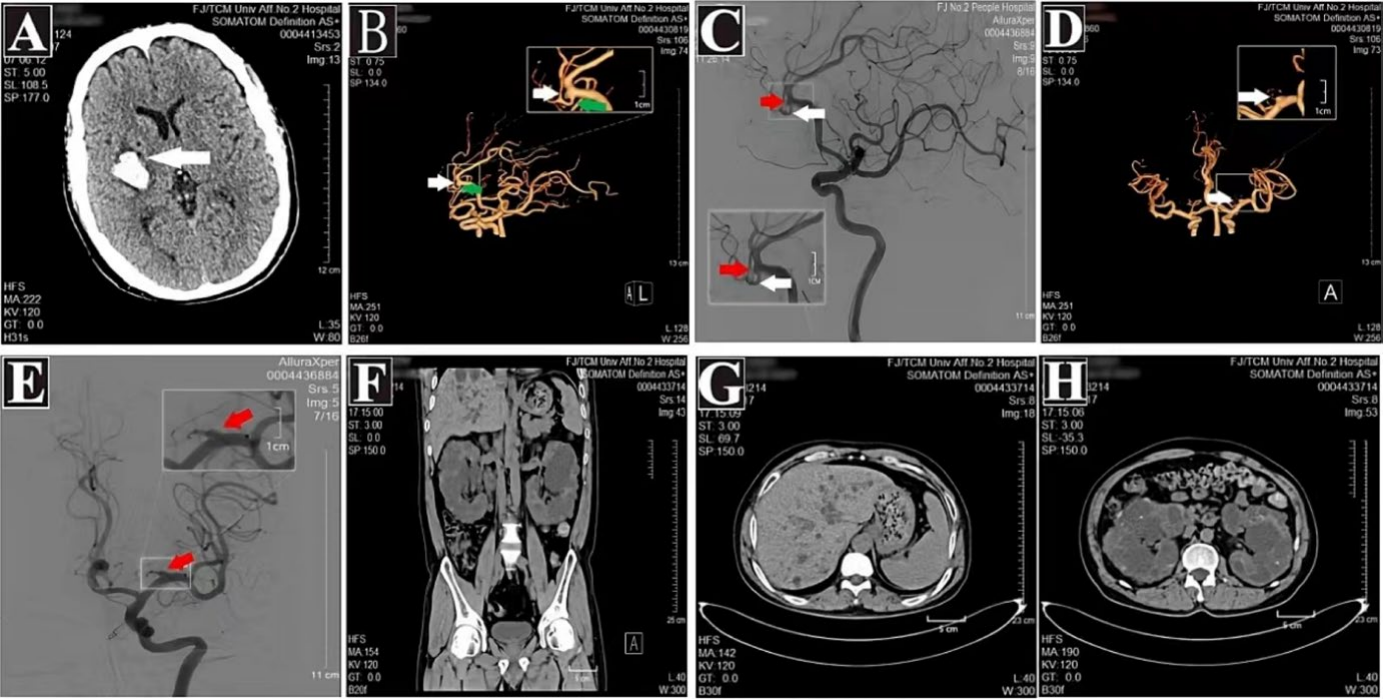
CT and CTA and digital subtraction angiography (DSA) of the second brother of proband II:4. (A) Cerebral hemorrhage in the right basal ganglia area; (B) CTA white arrow indicates anterior cerebral artery aneurysm, the green arrow indicates the callosal artery emerging from the side wall of the aneurysm; (C) DSA, the red arrow indicates anterior cerebral artery aneurysm, and the white arrow indicates the callosal artery emerging from the side wall of the aneurysm; (D) CTA, the white arrow indicates a middle cerebral artery aneurysm; (E) DSA, red arrow indicates a middle cerebral artery aneurysm; (F) Abdominal CT showing a polycystic liver and polycystic kidney; (G) Axial CT showing a polycystic liver; (H) Axial CT reveals polycystic kidney disease.
